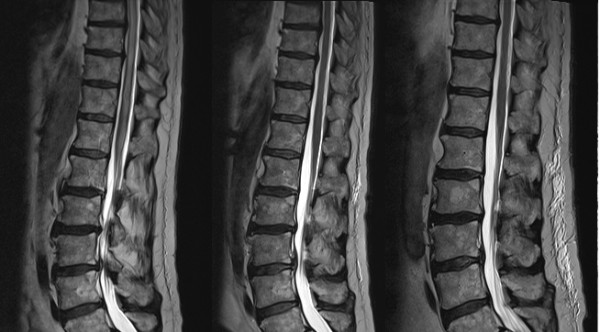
Chronological MRI images of lumbar spine - November 2008/December 2008/May 2009. In figure 1 the lumbar spine is most clearly affected in the first image (November 2008). The images show increased signal within the substance of the spinal cord during episodes of active vasculitis, with some subtle expansion of the cord.
